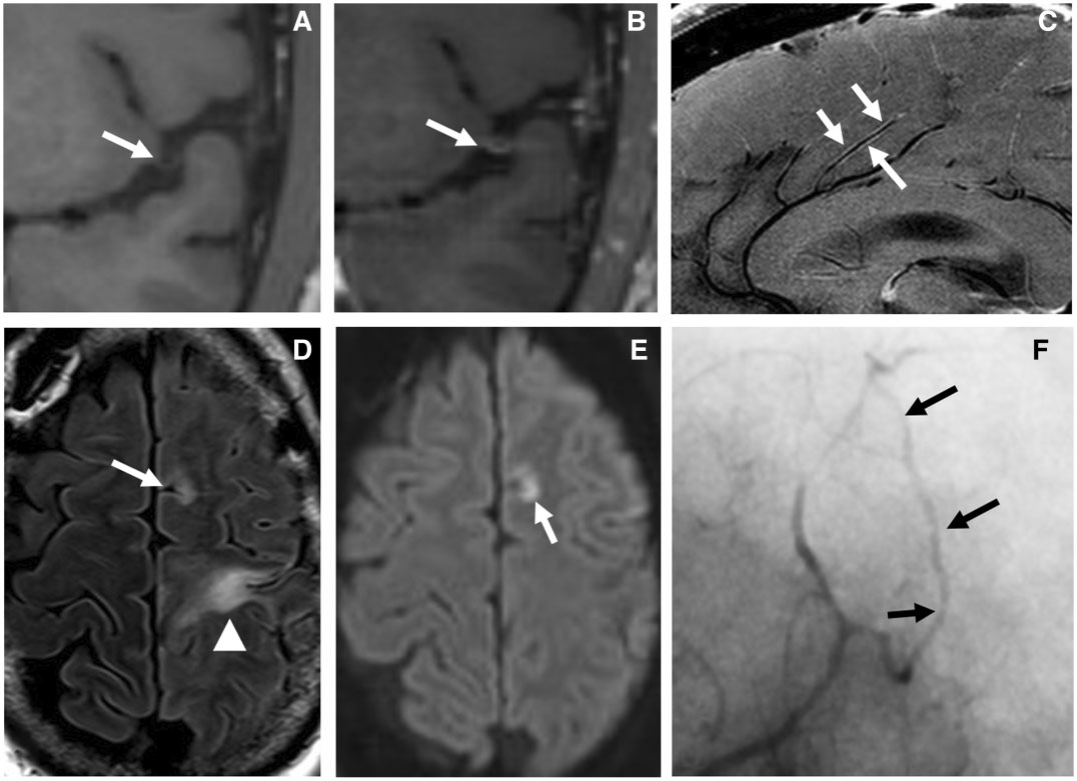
Radiographic findings of primary CNS vasculitis. (A, B) Pre- (A) and post-gadolinium (B) T1 SPACE high-resolution MR-VWI shows circumferential vessel wall enhancement (B, arrow) in a patient with suspected CNSV. (C) 7 T MR-VWI following gadolinium administration shows diffuse pericallosal artery branch wall enhancement in biopsy-proven CNSV (arrows). (D) FLAIR MRI showing evolving signal changes from parenchymal insults of varying ages, including subacute (arrowhead) and acute (arrow). (E) diffusion-weighted MRI in the same patient as (E) shows that the paramedian frontal insult is acute. (F) Digital subtraction angiography in the same patient showing subtle areas of distal anterior cerebral artery territory beaded luminal irregularity. CNSV: CNS vasculitis; FLAIR: fluid-attenuated inversion recovery; VW: vessel wall
